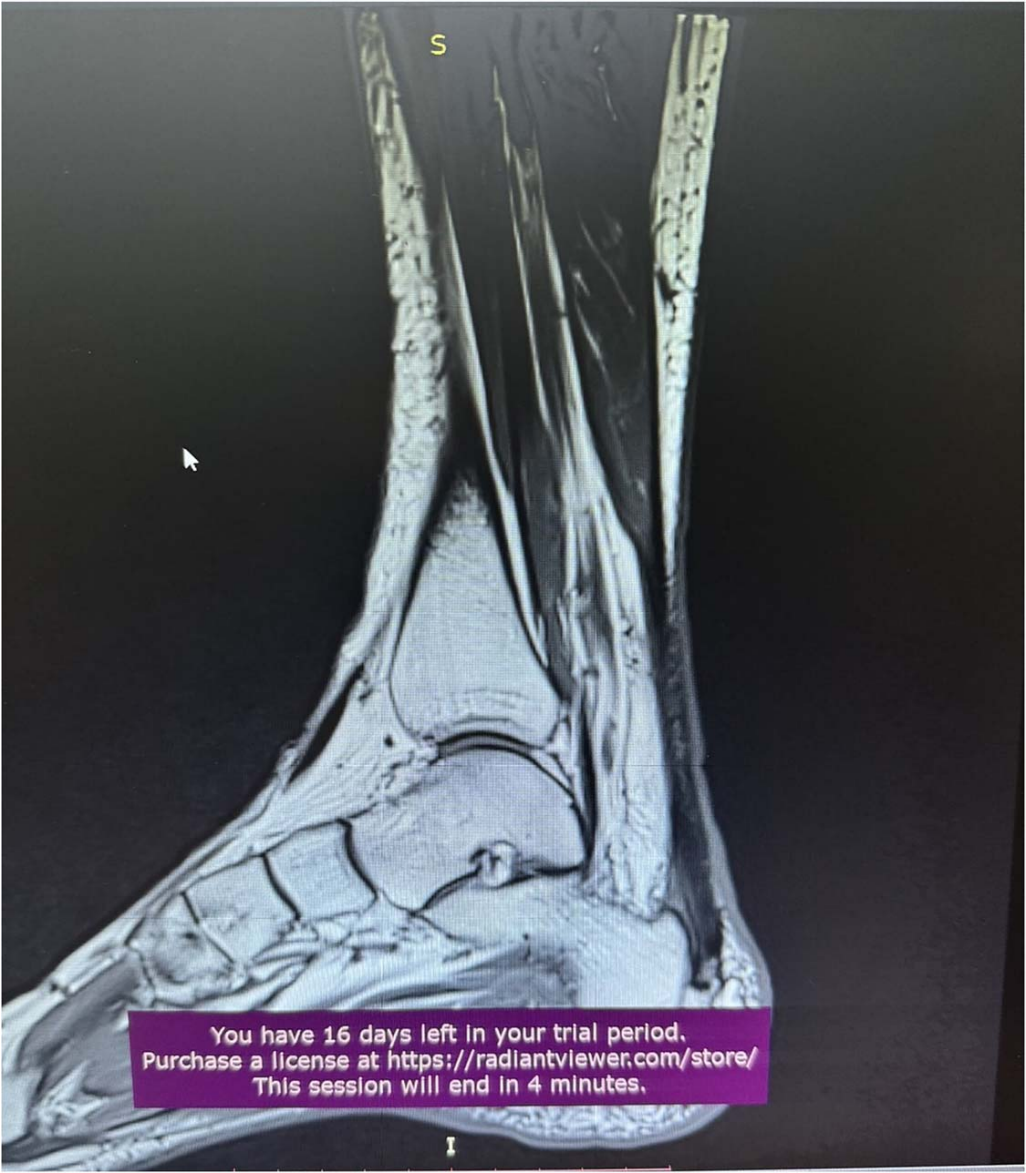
MRI of Achilles tendinopathy.
